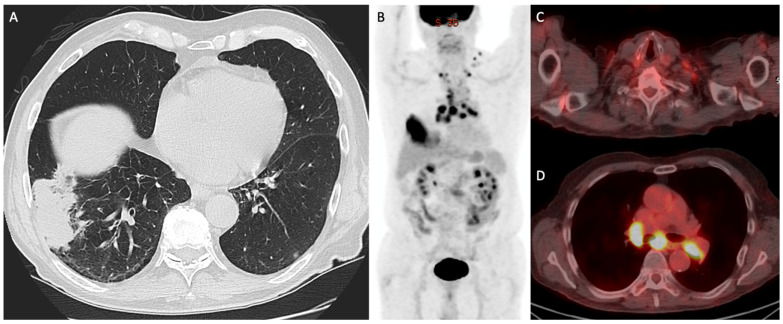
Demonstrates a 68-year-old man with biopsy-proven right lung adenocarcinoma (Stage: T3N3M0). (A) Axial CT showing a mass in the lateral right lower lobe, abutting adjacent pleura, with biopsy demonstrating adenocarcinoma. (B) ^18^F-FDG-PET/CT maximum-intensity projection and (C,D) fused images showing multiple enlarged, avid ipsilateral and contralateral mediastinal and supraclavicular lymph nodes, suspicious for metastases, with the left supraclavicular node subsequently biopsied confirming malignancy.
